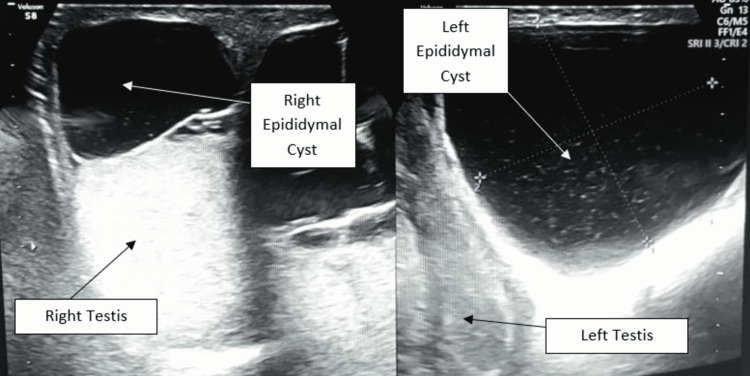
Scrotal ultrasonography images showing two large cystic swellings arising from both epididymal heads of size measuring 45 X 30 mm on the right and 100 X 70 mm on the left, with both testes normal in size, shape, and location.
